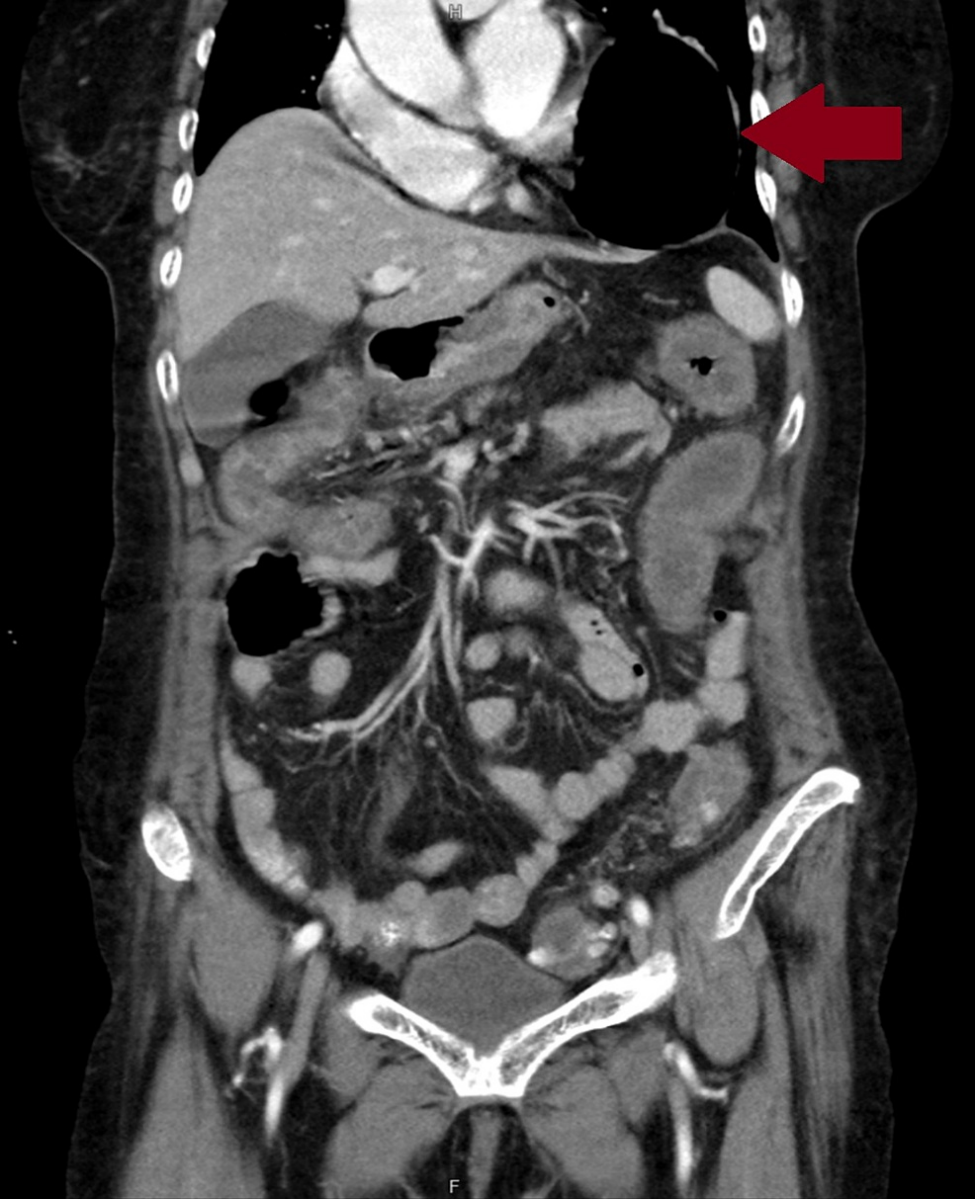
CT scan The red arrow points to the hiatal hernia compressing the heart
